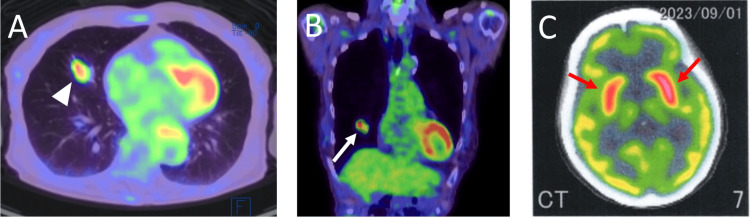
18F-fluoro-2-deoxy-D-glucose (FDG)-positron emission tomography (PET)-CT scan (A, B) Radioactivity is accumulated in the nodule in the middle lobe of the right lung (A, arrowhead; B, arrow). (C) There is slight hypermetabolism in the striatum of both hemispheres (red arrows).
